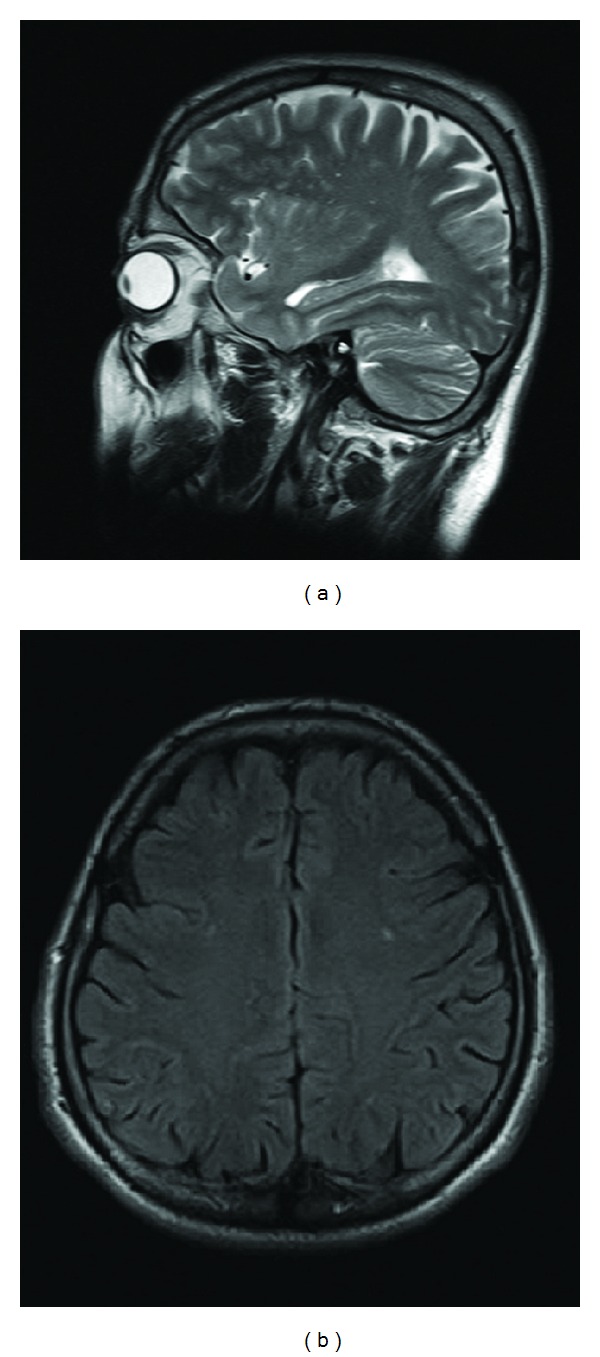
(a) T2-weighted sagittal and (b) axial flair images revealed the presence of small hyperintense pericallosal lesions and supraventricular white matter lesions.
